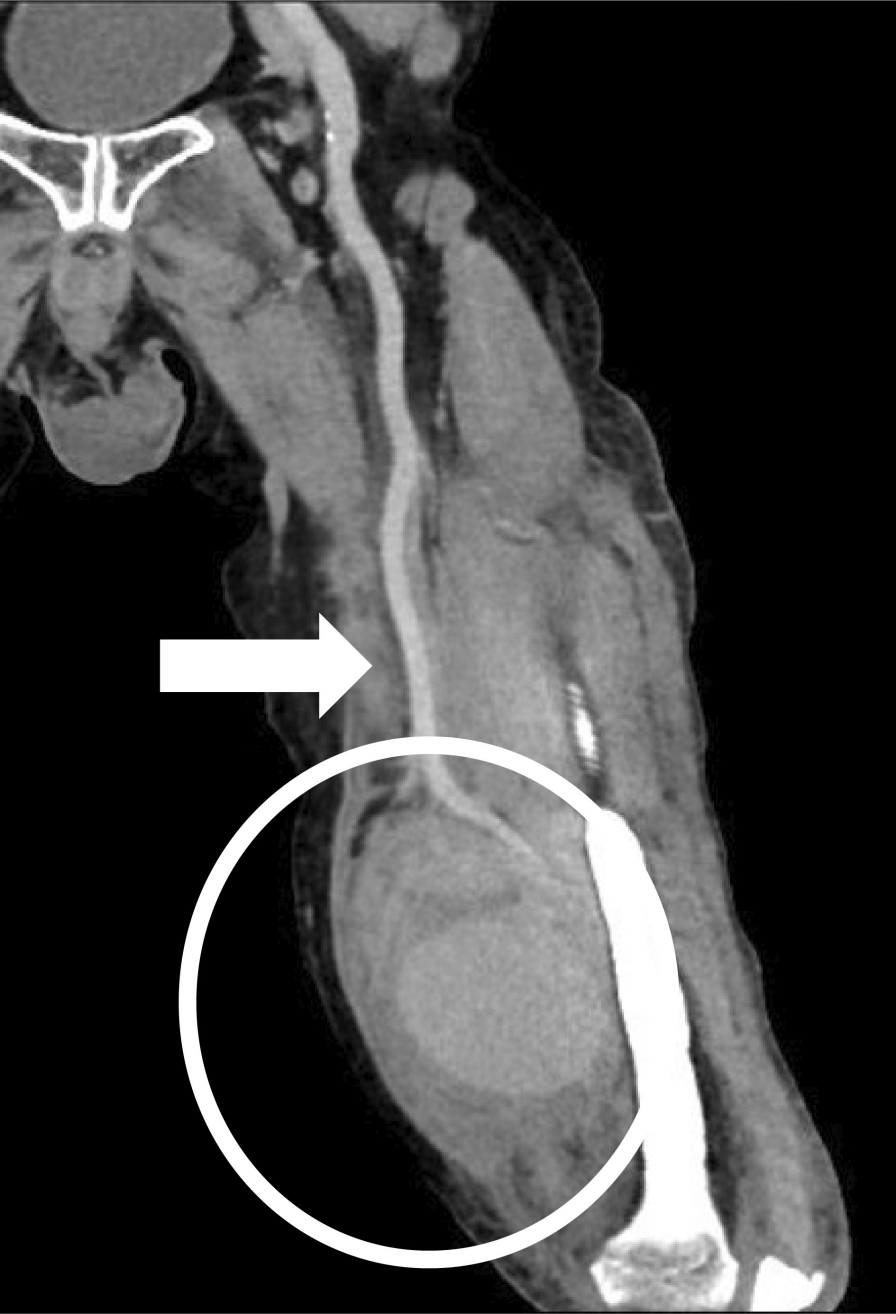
Preoperative contrast‐enhanced CT image of a 78‐year‐old man with superficial femoral artery pseudoaneurysm. The white arrow points to the left common femoral artery. Leakage of contrast medium and a hematoma can be seen (white circle).
